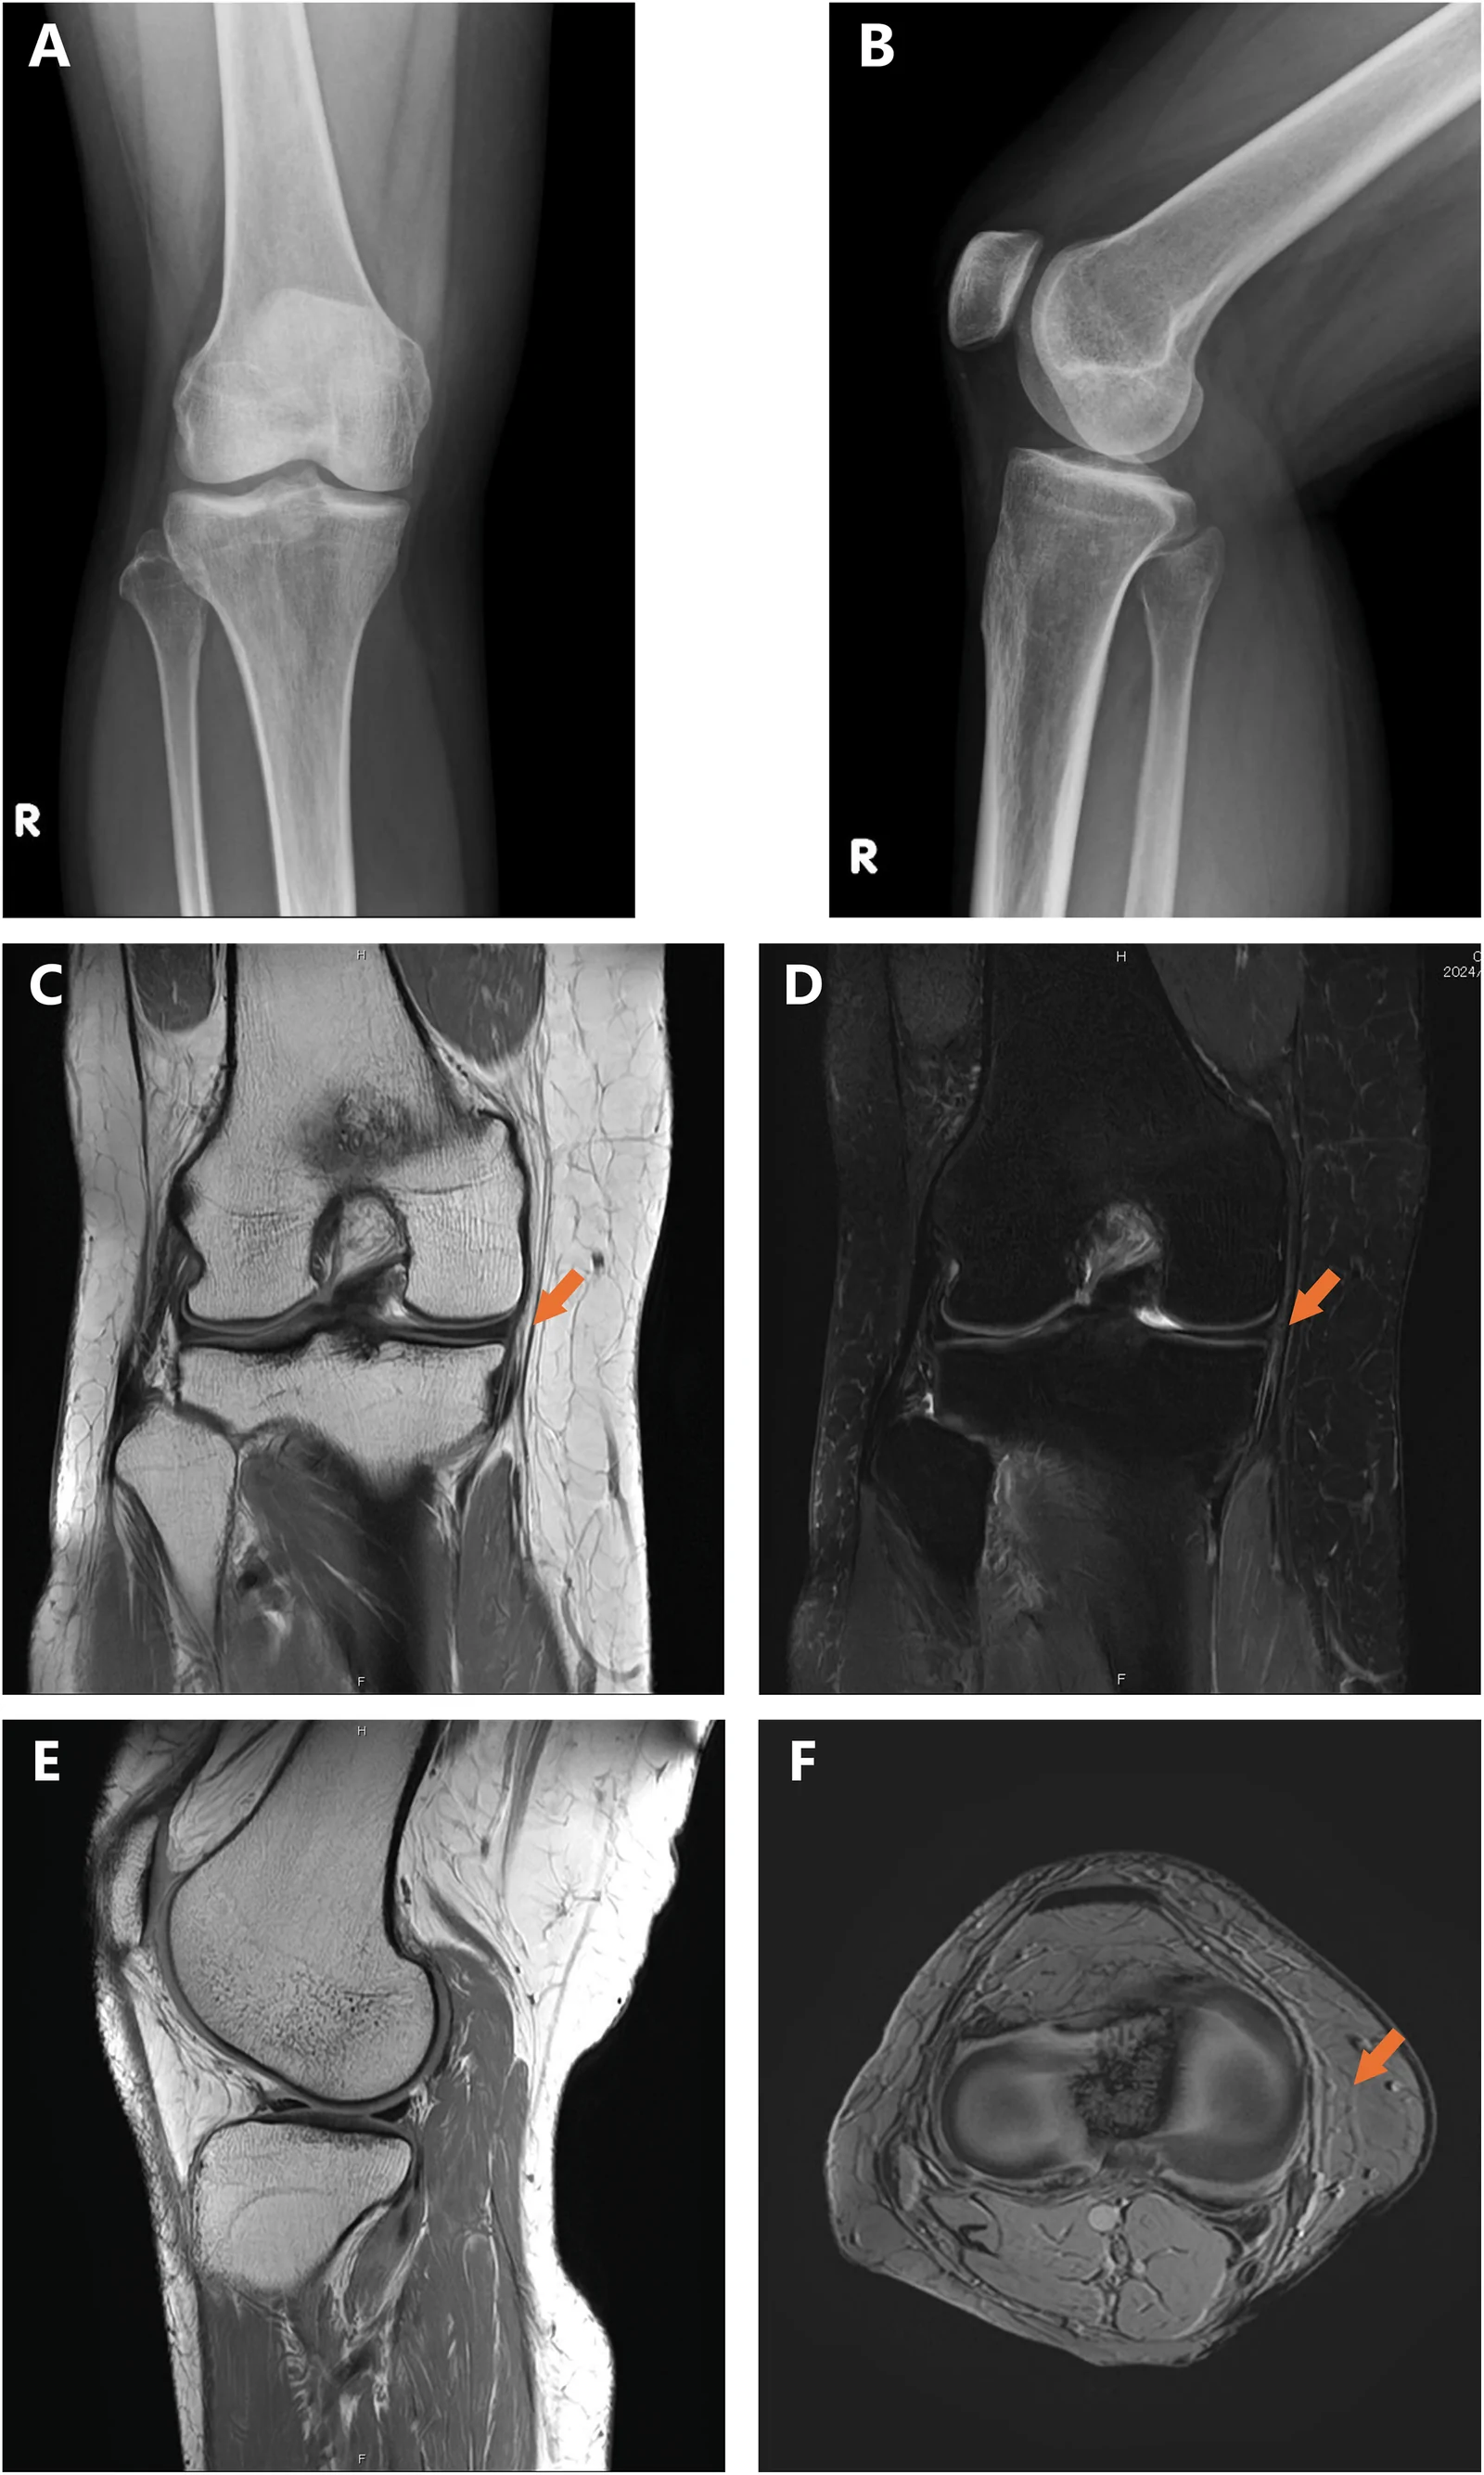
Preoperative imaging of the right knee. (A) Anteroposterior radiograph. (B) Lateral radiograph. (C) Coronal proton density-weighted image (PDWI) through the midbody of the medial meniscus and the adjacent meniscocapsular region. (D) Coronal T2-weighted fat-saturated image at the same level. (E) Sagittal proton density-weighted image through the central medial compartment near the medial tibial plateau. (F) Axial T2*-weighted image at the level of the medial meniscus and the adjacent meniscocapsular region. No definite meniscal tear or reproducible medial meniscocapsular lesion is identified on the preoperative images. Orange arrows indicate the approximate anatomic region that later corresponded to the arthroscopic valley-like concavity. However, no discrete abnormality is identifiable on MRI.
